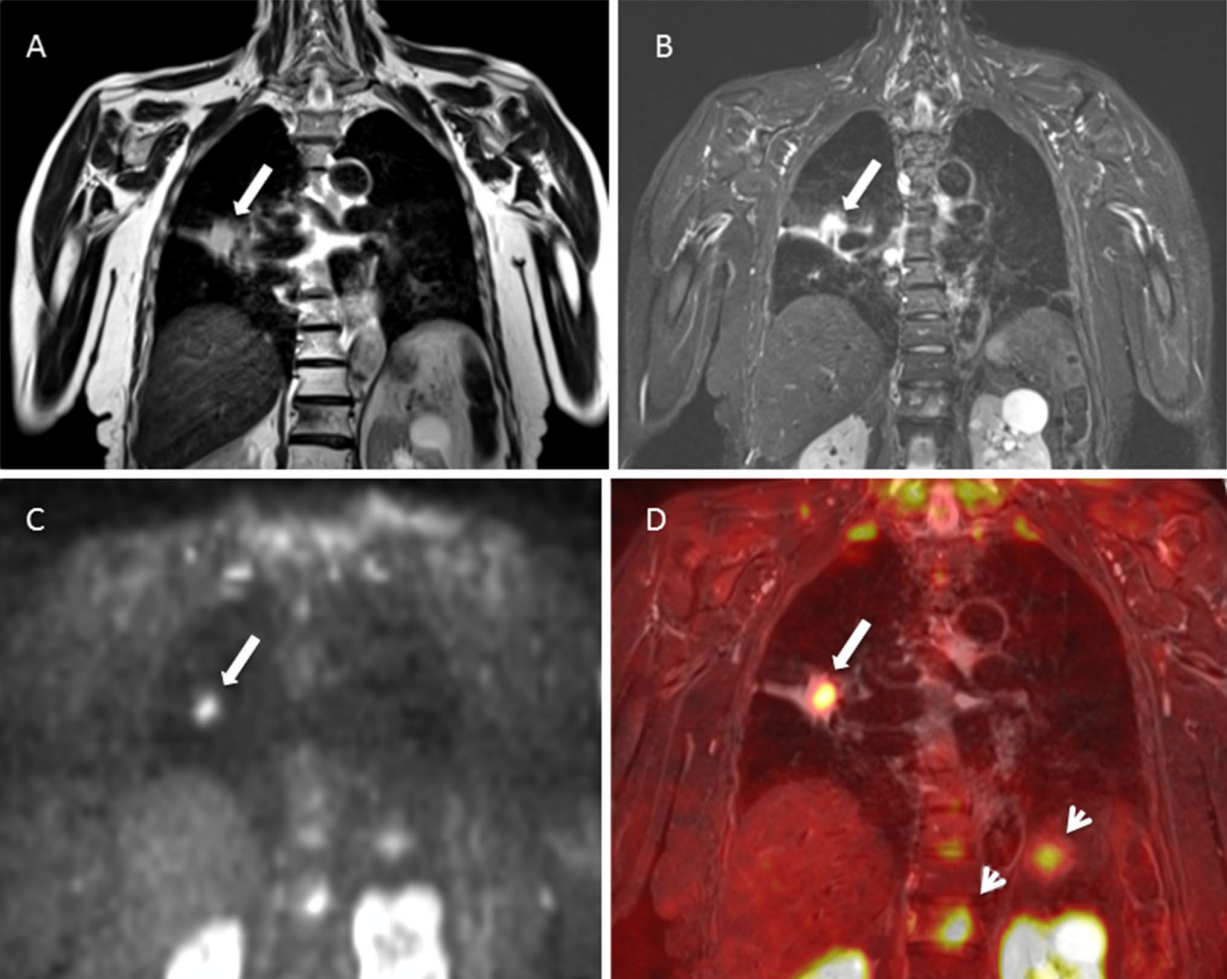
MRI in patient with lung adenocarcinoma. a T2-weighted and b STIR sequences. A nodule in the right lung parenchyma can be identified, which is contact and also thickens the minor fissure with associated atelectasis (arrow). c High b-value (1000 s/mm^2^) DWI shows increased signal of the pulmonary nodule (arrow), without high signal in the minor fissure consistent with a malignant origin and lack of fissure involvement. d Fused image of coronal T2-weighted image and DWI better depicts the nodule (arrow) and metastatic lesions at L1 vertebral body and left adrenal gland (arrowheads) with advantage over morphological sequences (a, b)
